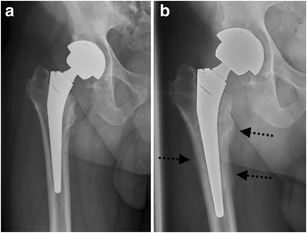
a AP radiograph, 1 month postoperatively. Cementless total hip arthroplasty. Normal postoperative findings. b AP radiograph of the same patient, 3 months postoperatively. Periosteal reaction in Gruen zone 2/5/6/7 (*arrows*): proven case of infection
